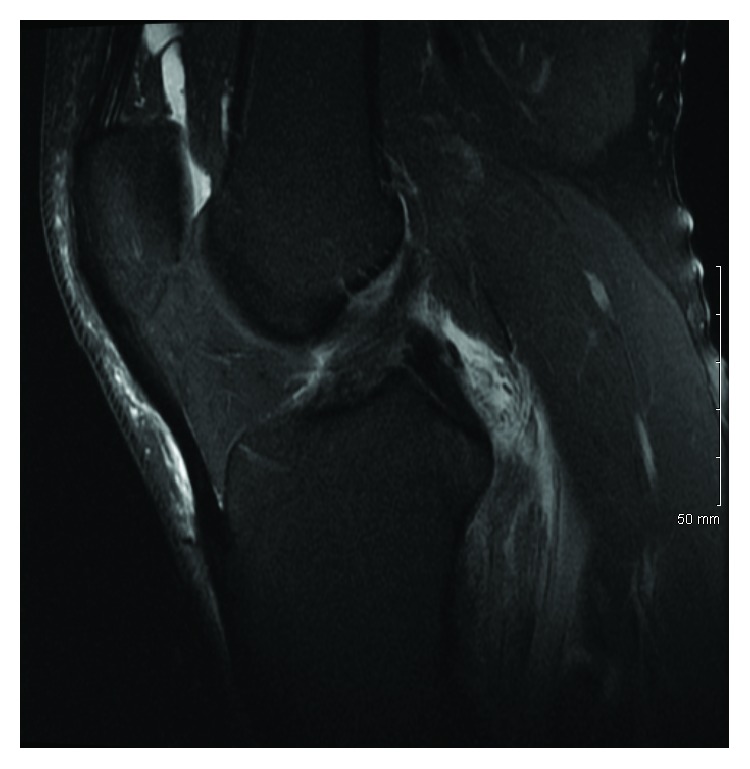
A T2 sagittal MRI of the patient's left knee demonstrating a grade II partial thickness tear of the anterior cruciate ligament.
